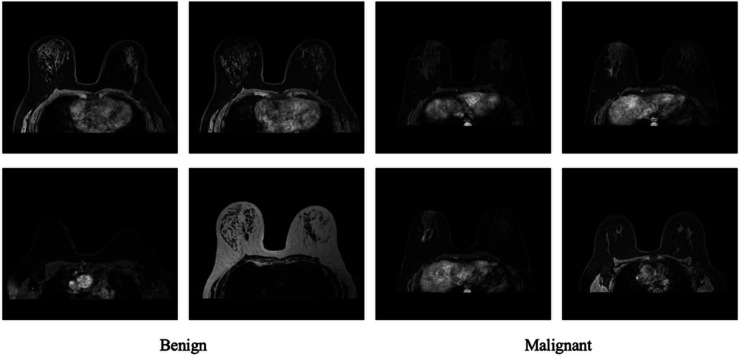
Collection of breast MRI scans, categorized into Benign and Malignant cases.
